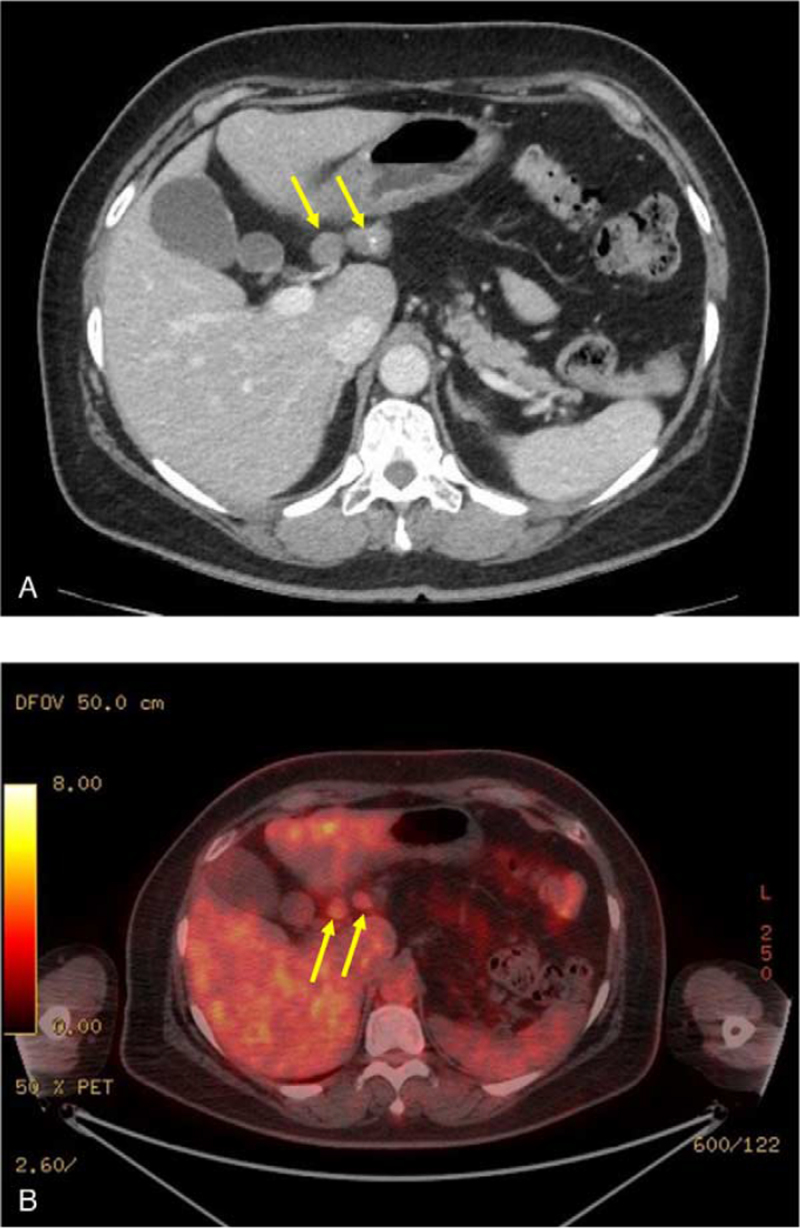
Lymph node recurrence, as observed in July 2021. (A) Computed tomography image showing two enlarged lymph nodes of size 15 and 20 mm. (B) Positron emission tomography scan image showing two hypermetabolic lymph nodes.
